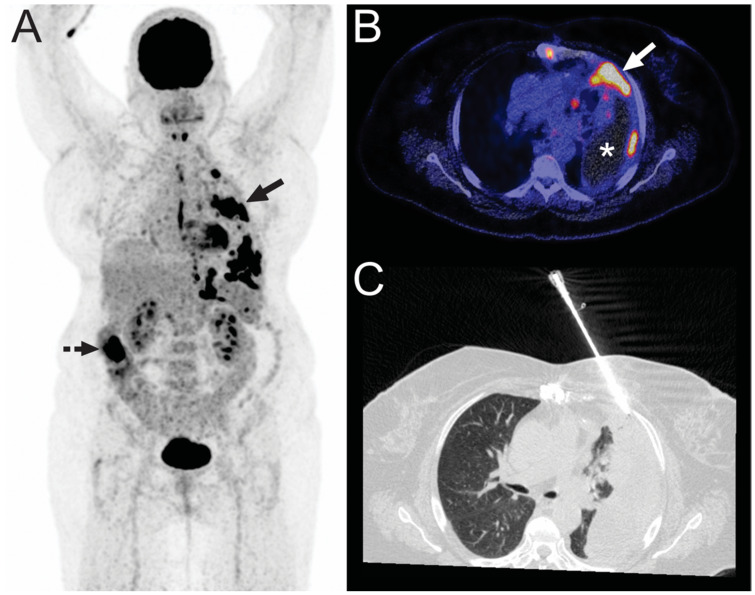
A 70-year-old woman with a known left-sided pleural effusion and a suspicion of lung cancer based on previous chest radiography and CT (not shown) was referred for FDG-PET. FDG-PET (A) showed multiple pleural lesions (continuous arrow), also demonstrated at fused FDG-PET/CT ((B), arrow), along with a pleural effusion ((B), asterisk). FDG-PET also showed an FDG-avid area in the ascending colon, which was interpreted as either physiological or due to diverticulitis or colitis by the attending nuclear medicine physician. CT-guided biopsy was ordered by the pulmonary oncologist to confirm the diagnosis of lung cancer and mutation analysis. CT image (C) shows the biopsy needle just before tissue sampling of one of the pleural lesions. Pathologic examination revealed intestinal adenocarcinoma and no lung cancer. Colonoscopy was performed, which demonstrated a tumor in the ascending colon that proved to be a well- to moderately-differentiated adenocarcinoma after biopsy.
